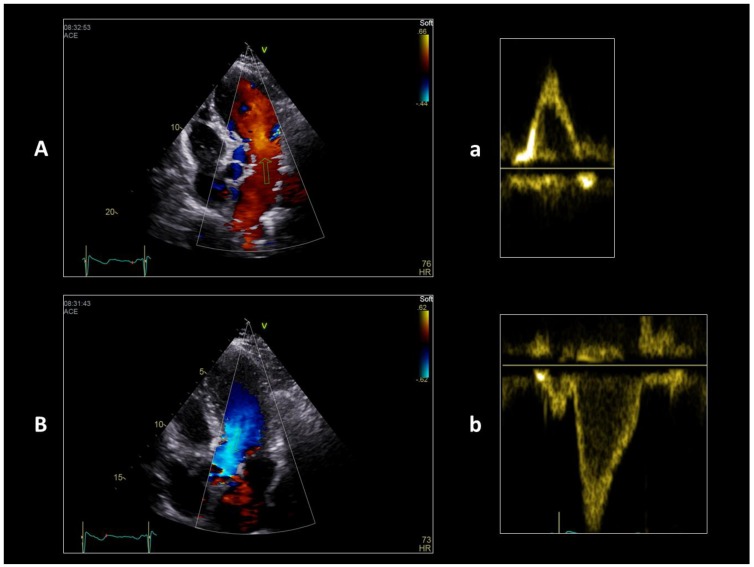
Echo color Doppler (A,B) and Pulsed Doppler (a,b) study of diastolic (A,a) and systolic (B,b) intra-ventricular flows in a healthy volunteer. The color homogeneity and the black areas under the pulsed curves indicate a laminar flow.
